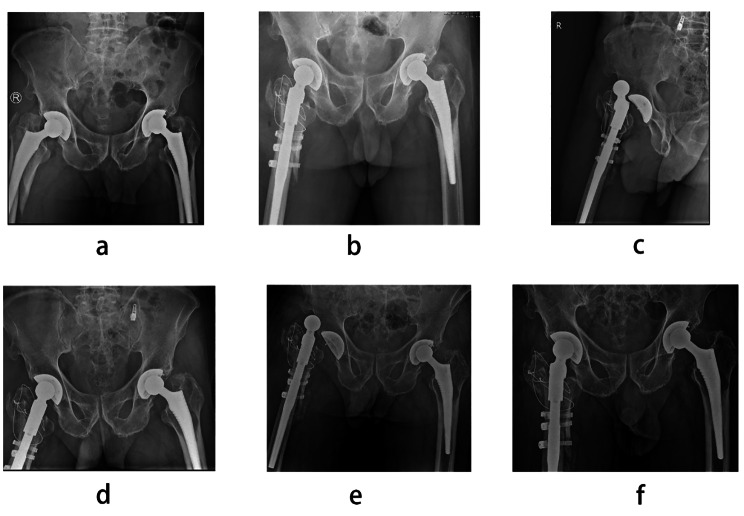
a A 73-year-old male who sustained a Vancouver type B2 fracture 2 years after THA due to femoral head necrosis. b Patient underwent revision surgery with a modular prosthesis, but postoperative pelvic anteroposterior X-ray showed that FO was not restored. c and d The patient had hip joint dislocation 1 month after revision surgery and received closed reduction. e and f The patient had redislocation of the hip joint 2 weeks after closed reduction and received closed reduction
